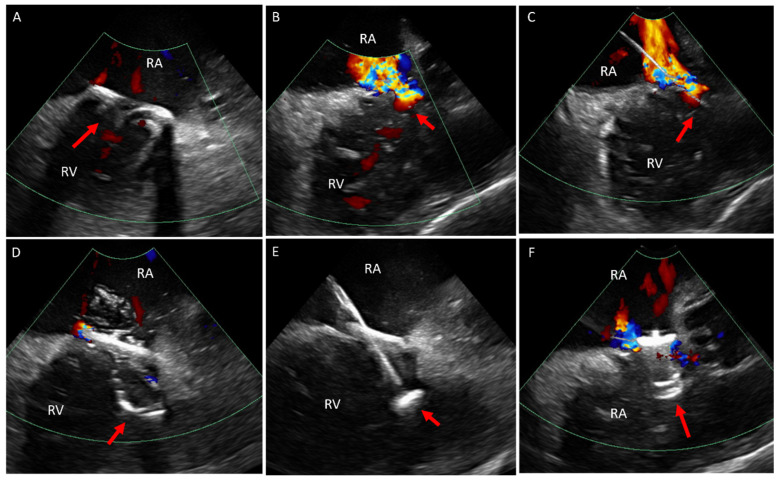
ICE catheter within the right atrium. (A) Color Doppler at the tricuspid valve (arrow) showing no intravalvular regurgitation. (B) Color Doppler when rotating the ICE catheter medially shows severe medial paravalve regurgitation (arrow). (C) Color Doppler at the tricuspid valve annulus showing a wire crossing the paravalve area. (D) Color Doppler while balloon (arrow) sizing and occluding the paravalve leak showing only minimal residual leak. (E) Showing the closure device attached to a cable across the defect and in the RV. (F) Color Doppler after deployment and release of the closure device in the paravalve leak area showing only mild residual leak. ICE, intracardiac echo; RA, right atrium; RV, right ventricle.
